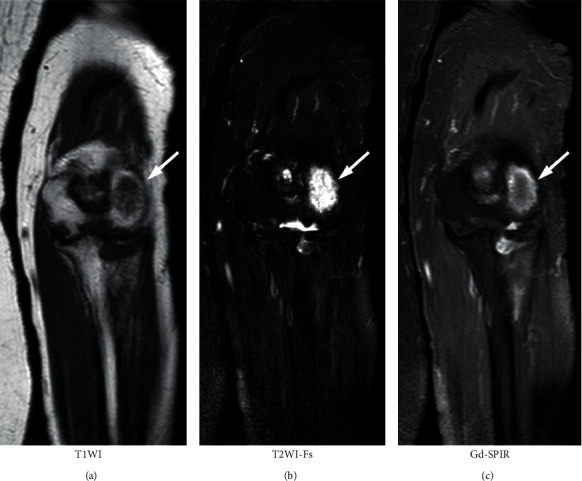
Magnetic resonance image of the left elbow, 3 years 5 months after the first visit to our department. (a) T1-weighted image (T1WI); (b) fat-suppressed T2-weighted image (T2WI-Fs); and (c) gadolinium-enhanced short T1 inversion recovery image (Gd-SPIR). The high-intensity area appeared from the peripheral cavity and internal low-intensity area became slightly brighter in T1WI. The peripheral high-intensity area was considered to demonstrate replaced bone marrow fat. The high-intensity area is noted in T2WI-Fs, but the size and signal strength decreased. In Gd-SPIR, the low-intensity area became slightly brighter, and the peripherally enhanced area seemed to be weaker (arrow).
